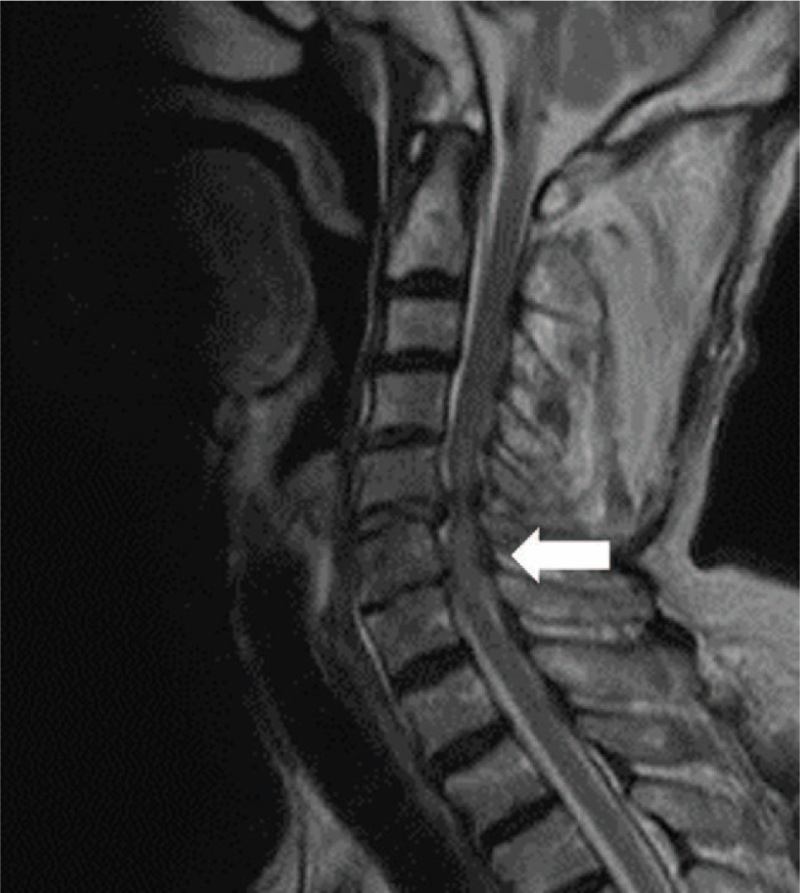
MRI (T2-weighted image). A high signal was found in the cervical spinal cord at the C5/6 level. MRI = magnetic resonance imaging.
